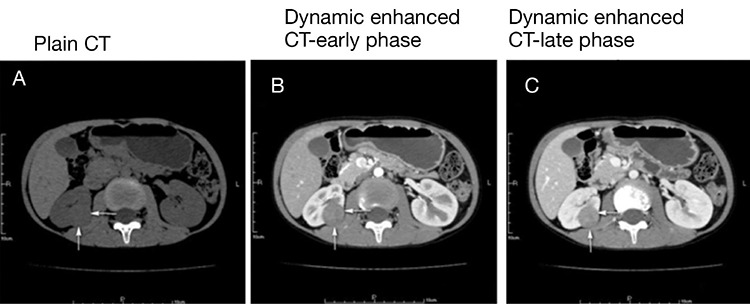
A-C. Computed tomography scan of juxtaglomerular cell tumor. Representative images of plain computed tomography scan (A), dynamic enhanced computed tomography scan at the early phase (B) and late phase (C) were shown.
